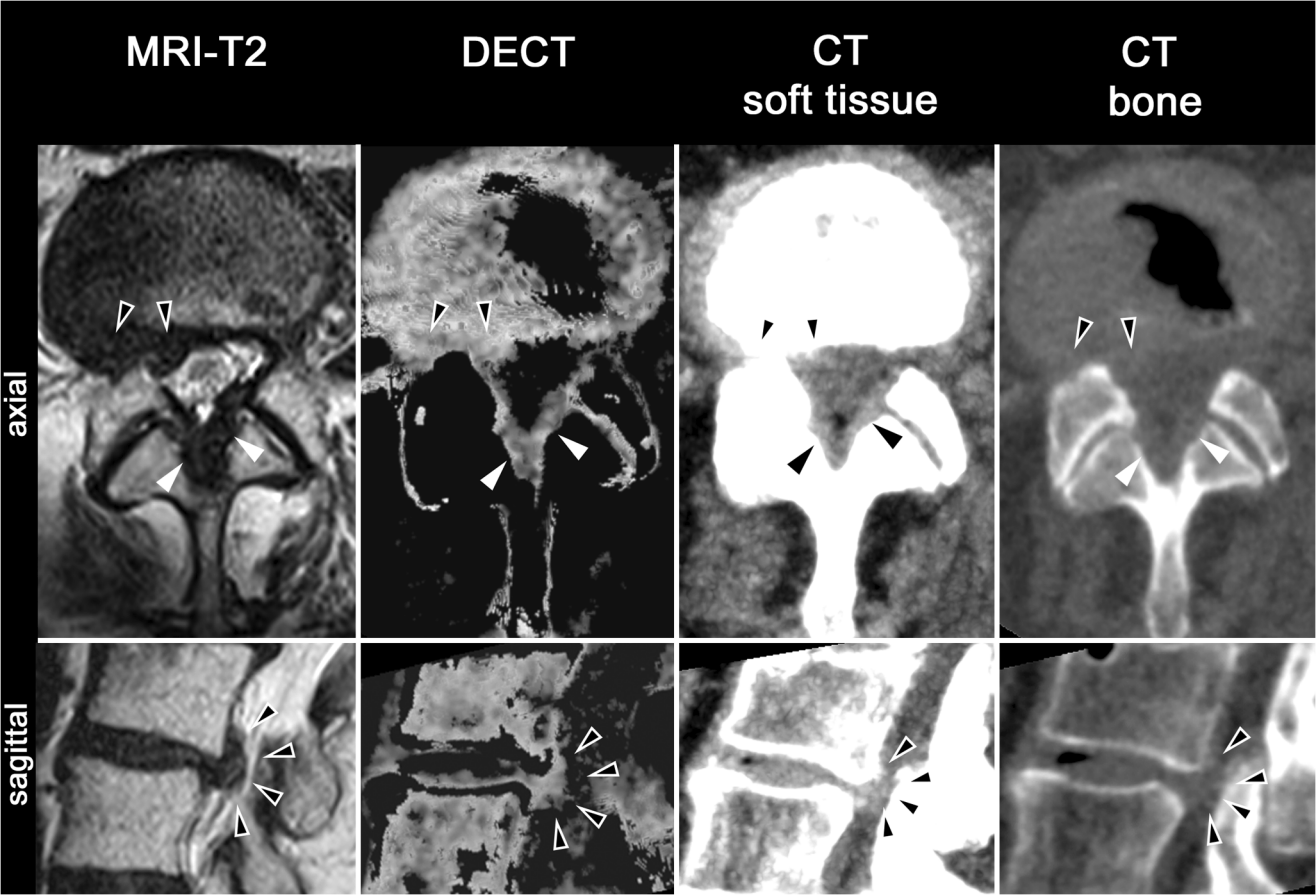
Representative example of a scored imaging dataset. Axial and sagittal T2w MR images, collagen-reconstructed DECT images, and conventional (DE-)CT images in soft tissue and bone window of a patient with focal herniation with extrusion and disk migration into right subarticular, foraminal, and extraforaminal areas. Arrowheads: displaced disk material; filled arrowheads: ligamentum flavum
